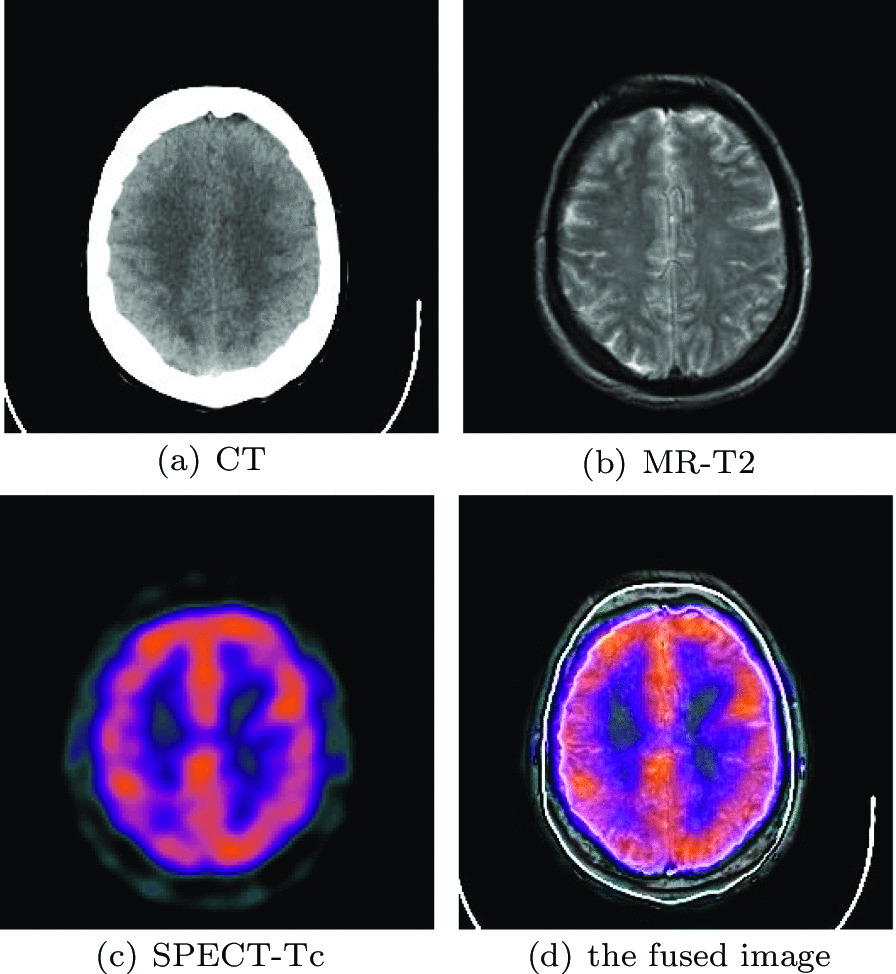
Tri-modal fusion of magnetic resonance imaging, computed tomography, and single-photon emission computed tomography slices. Best viewed on screen
